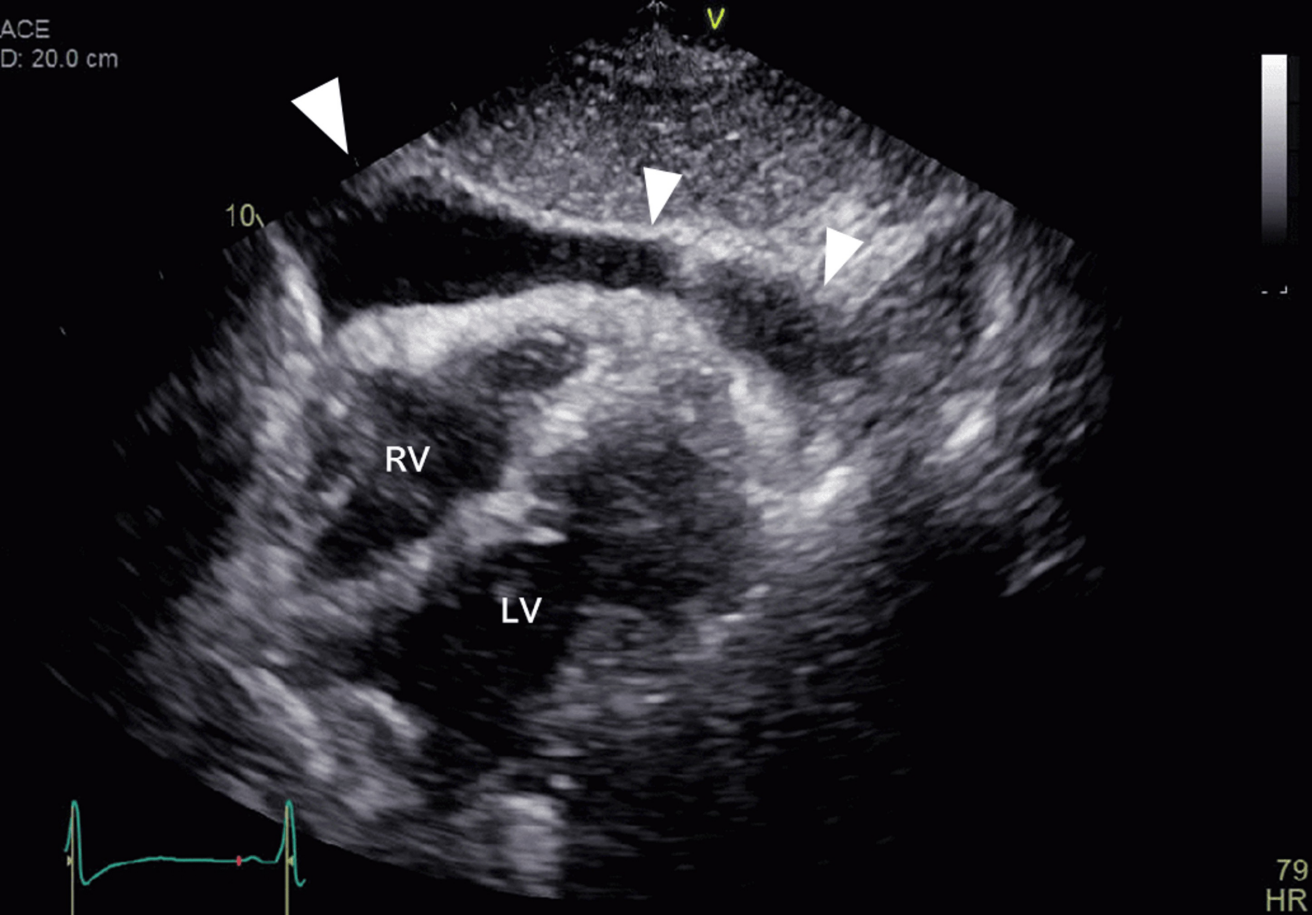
Echocardiographic image at the time of moderate pericardial effusion (in November 2024) On echocardiography, a moderate amount of pericardial effusion was predominantly located anterior to the right heart (white arrowhead), and right heart collapse was suspected RV: right ventricular; LV: left ventricular
